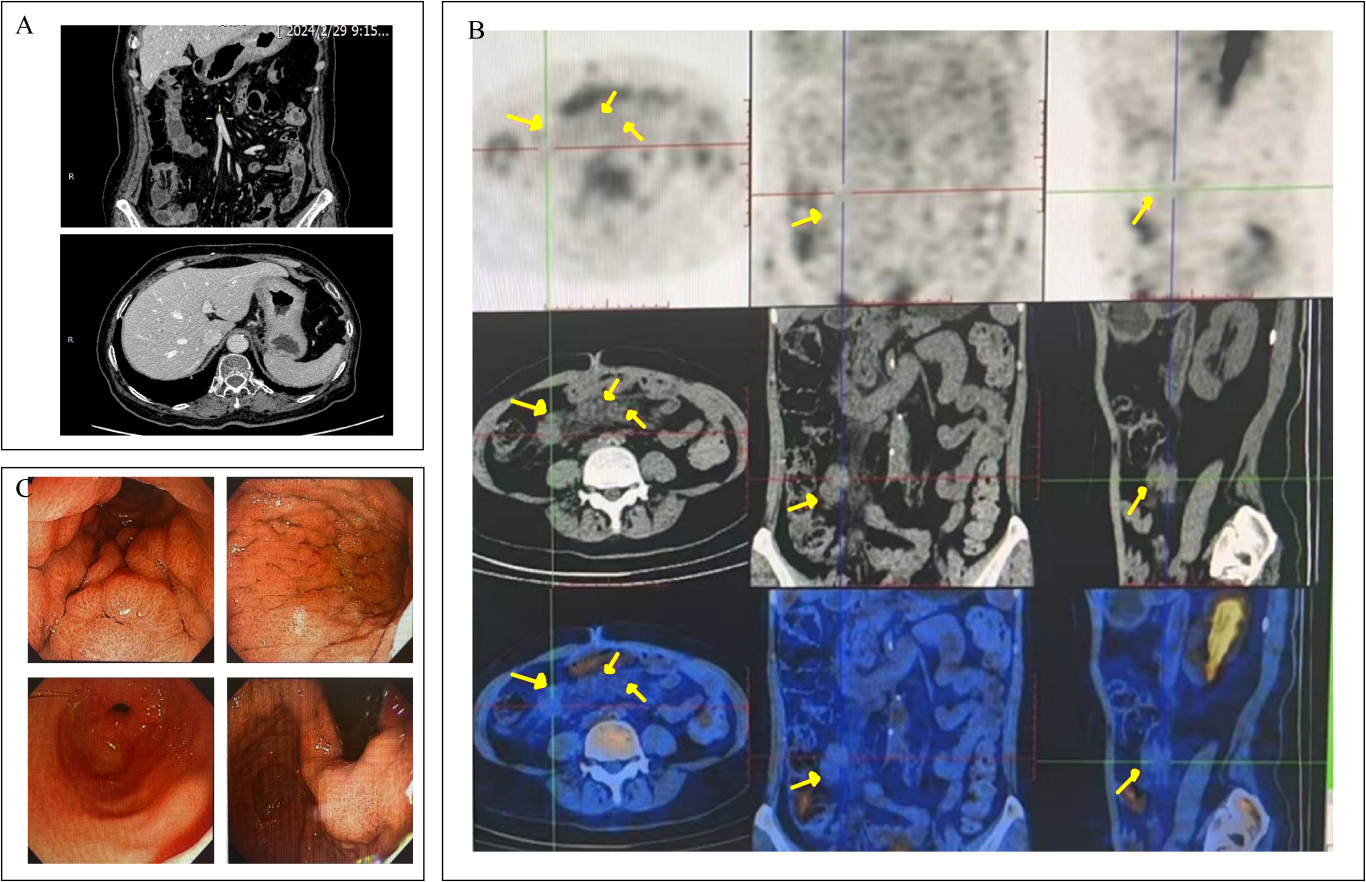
The CT, PET-CT and gastroscopic findings of the patient. (A) Contrast enhanced CT of the abdomen showed diffuse thickening of the gastric wall throughout the stomach. (B) PET-CT showed multiple hypermetabolic foci of mesenteric as well as retroperitoneal lymph nodes (locations marked with yellow arrows), which were considered to be metastases of the tumor. (C) Gastroscopy showed congested, edematous thickening of the gastric wall.
